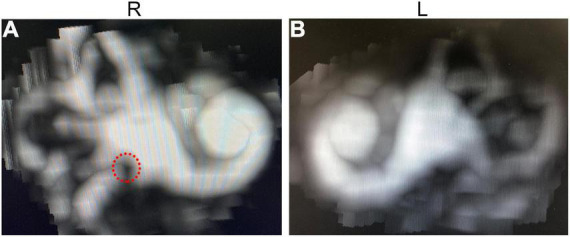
MIP images of MRI for III-1 following intravenous gadolinium (Gd) injection. (A) Image of the right ear. (B) Image of the left ear. The red dotted circle: showed distensible sacculus in the vestibular pool. R, right ear; L, left ear.
